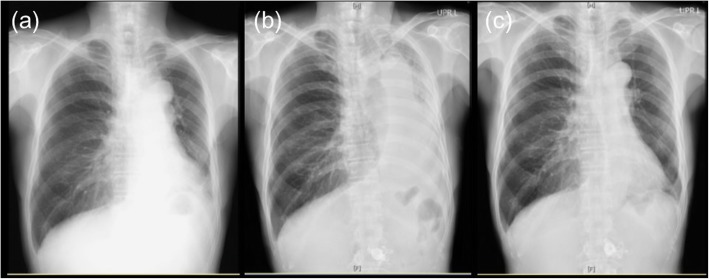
a Chest radiography performed 26 days before admission revealed no mediastinal shift; b chest radiography on the day of admission revealed a mediastinal shift and left atelectasis; c chest radiography performed 8 days after initiating chemoradiotherapy revealed no mediastinal shift
